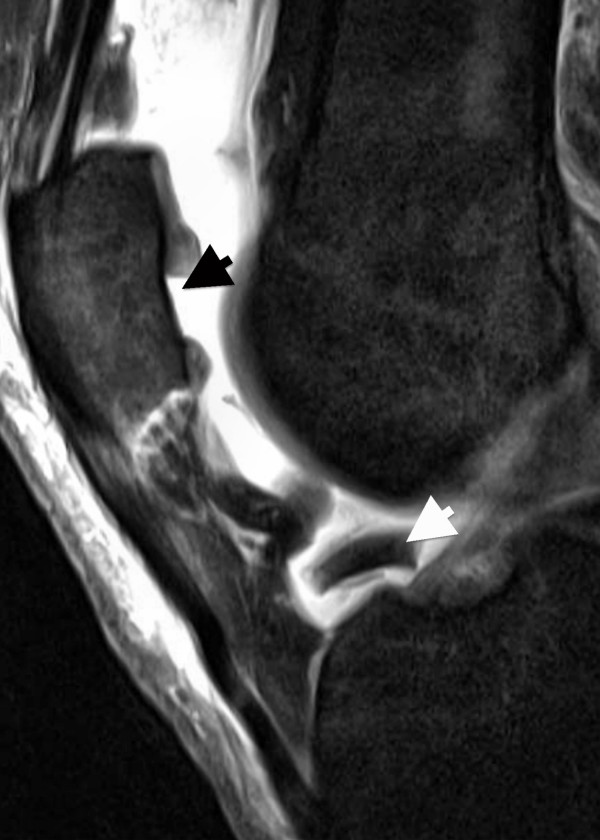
Saggital PD-weighted TSE MRI of the same patient depicted in Figure 5. A dislocated fragment lying in the intercondylar notch (short white arrow) corresponds to the full thickness cartilage defect at the central part of the patella (short black arrow).
